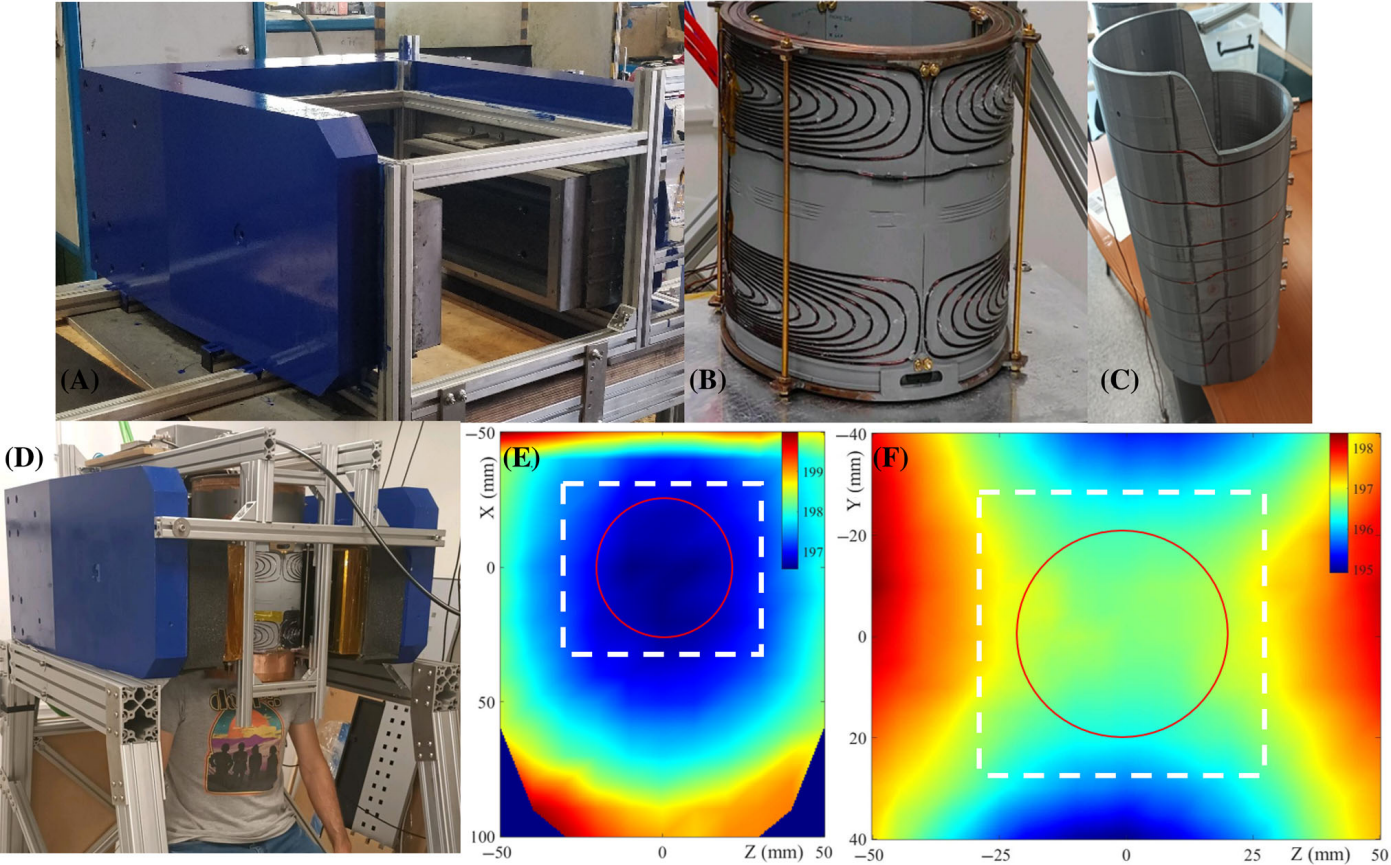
197 mT scanner designed for low‐field dental MRI. (A) Yoked magnet with shimming units. (B) Water‐cooled gradient system. (C) Radiofrequency excitation coil. (D) Fully assembled scanner. (E) Principal magnetic field $B_{0}$ in the xz plane (y=0). (F) Field in the yz plane (x=0). The square represents the (6 cm)
